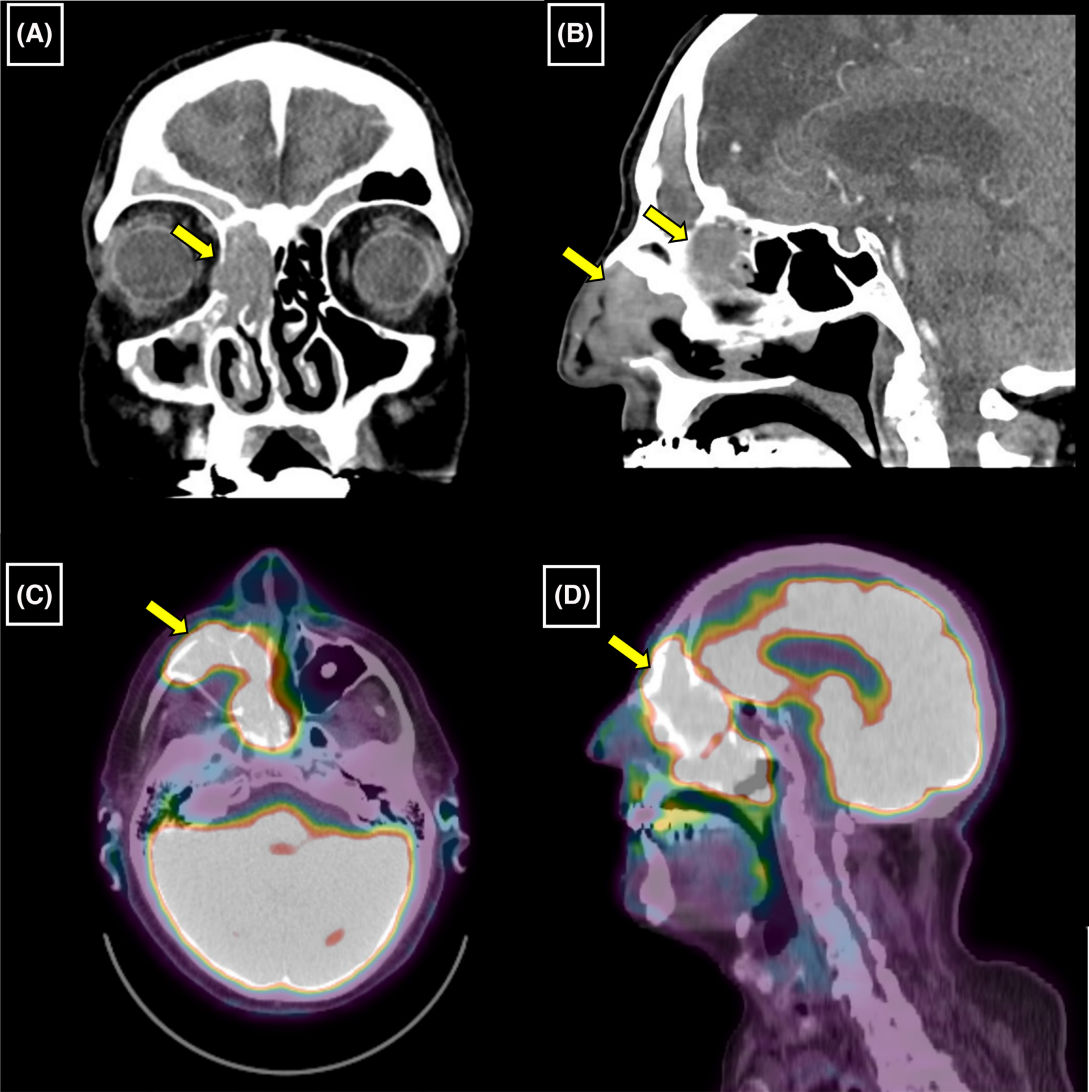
(A, B) Fast growing aggressive mass in the paranasal sinuses greater on the right with focal extension into the right orbit and focal disruption of the right cribriform plate. (C, D) Large intensely hypermetabolic infiltrative mass involving the right paranasal sinuses, right nasal cavity with septal defect and slight extension into the left nasal cavity, medial border of the right orbital cavity, left frontal sinus, right‐sided naso‐ and oropharynx, as described above (SUV max. up to 18.6).
